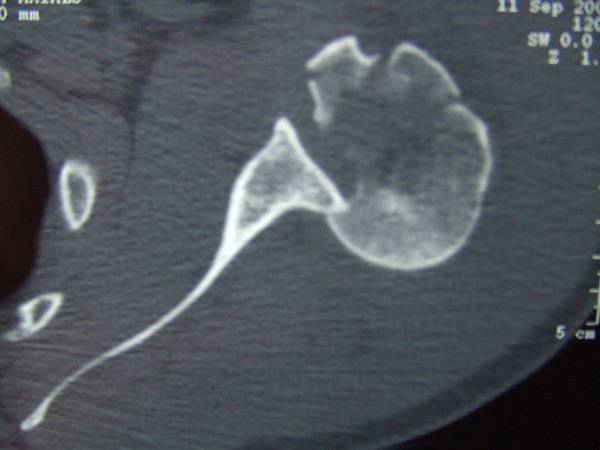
CT scan showing impacted humeral head with Hill-Sachs lesion and fractures of tuberosities.
